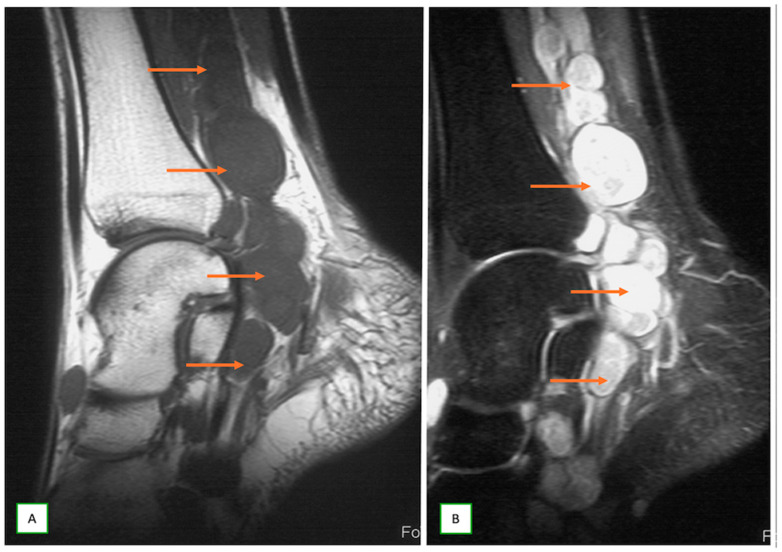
Multiple contiguous nerve sheath tumors of the tibial nerve in a case of schwannomatosis syndrome in a 37-year-old man. Sagittal T1 (A) and sagittal fat-saturated T2 (B) images show the multiple lesions (thin arrows) that appear low on T1 and high on T2-weighted images.
